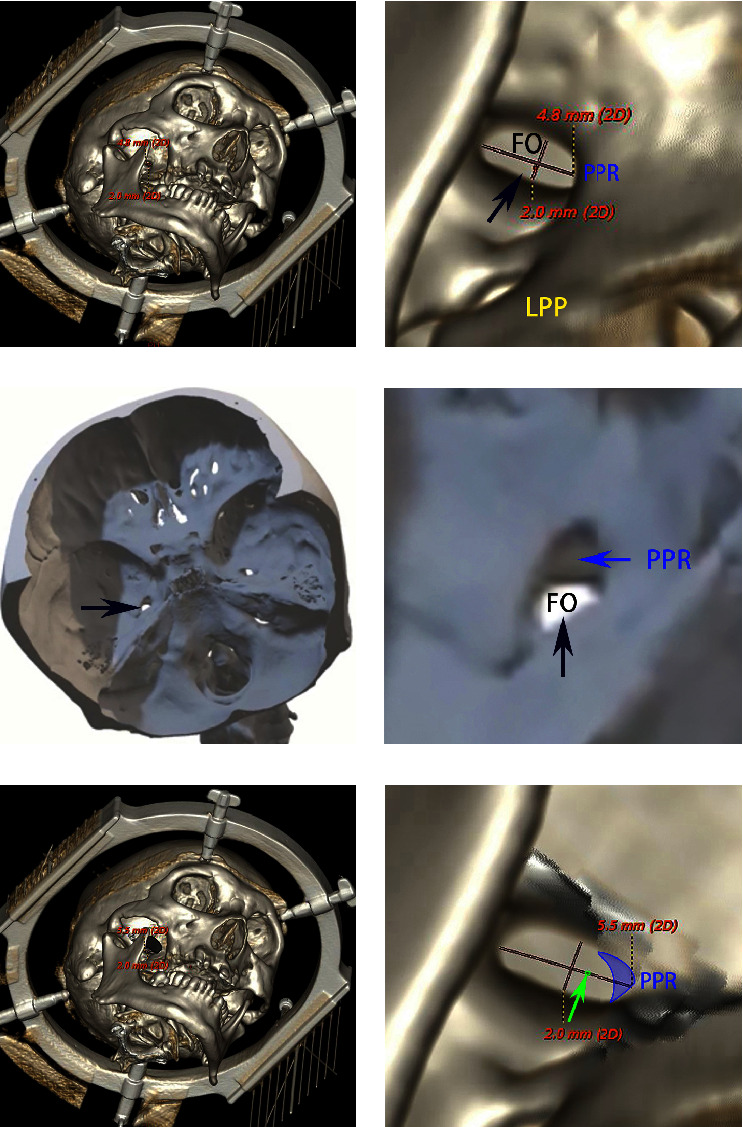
Three-dimensional computed tomography reconstruction images in the operational view. (a) The transverse diameter of the FO was 4.8 mm, and the anterior-posterior diameter was 2.0 mm. The image on the right shows a magnification of the FO from the left. (b) A 3D-printed model shows the shape of the FO and PPR from an interior view of the skull base. (c) We removed part of the PPR obstructing the FO through the mandibular notch, ensuring the integrity of the FO boundary. The transverse diameter was changed to 5.5 mm. The image on the right shows a magnification of the FO from the left.
